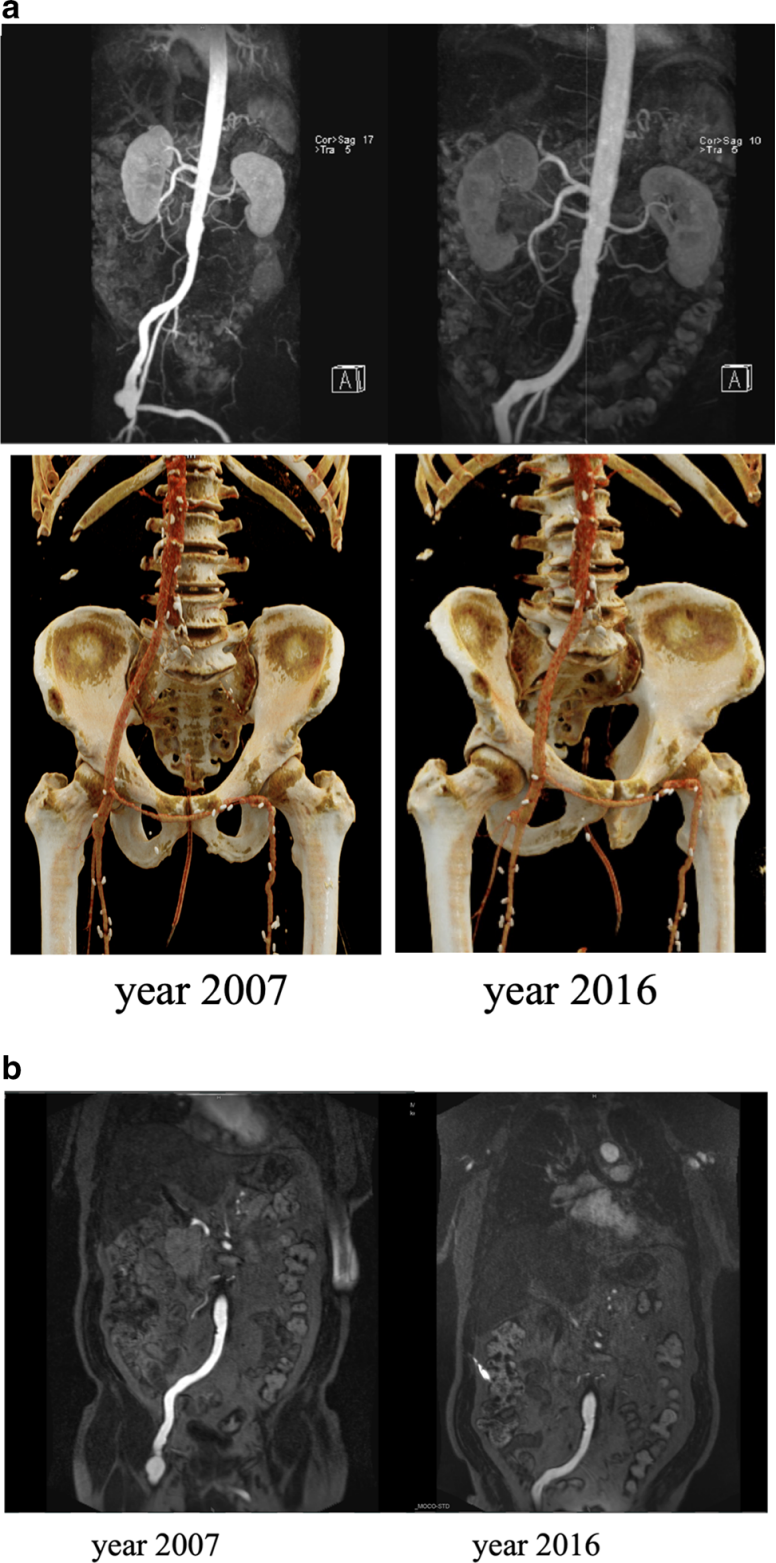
Aorto-iliac reconstruction with extra-anatomic femoro-femoral crossover bypass. Postoperative computed tomography angiography (CTA) scan with three-dimensional reconstruction two weeks after surgery a and after 9 years b. Proximal as well as distal anastomoses are elongated; however, the bypass is patent
